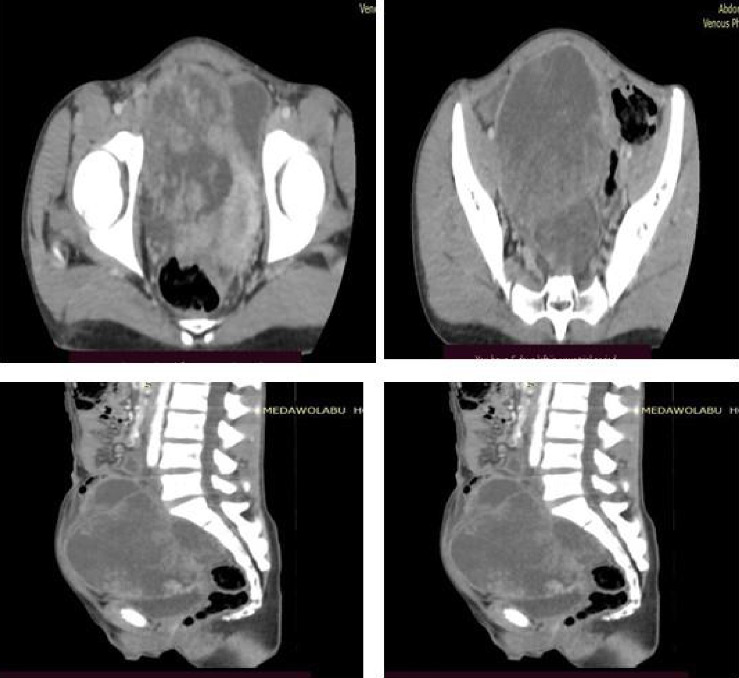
Contrast-enhanced computed tomography (CECT) scan demonstrating a giant, heterogeneously enhancing right adnexal mass with a strong suspicion of malignancy.
